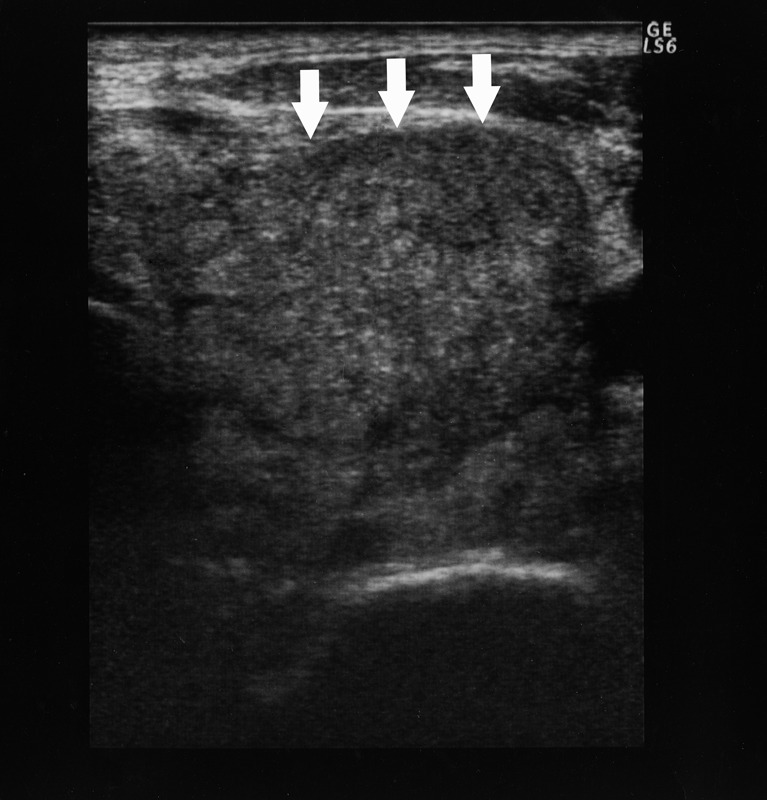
Ultrasonographic findings of the thyroid tumor. Ultrasonography reveals a 3.1 cm-sized tumor in the left lobe of the thyroid gland (arrows). It shows mosaic echogenecity and no calcification inside with a partially unclear border but no apparent spicular formation.
